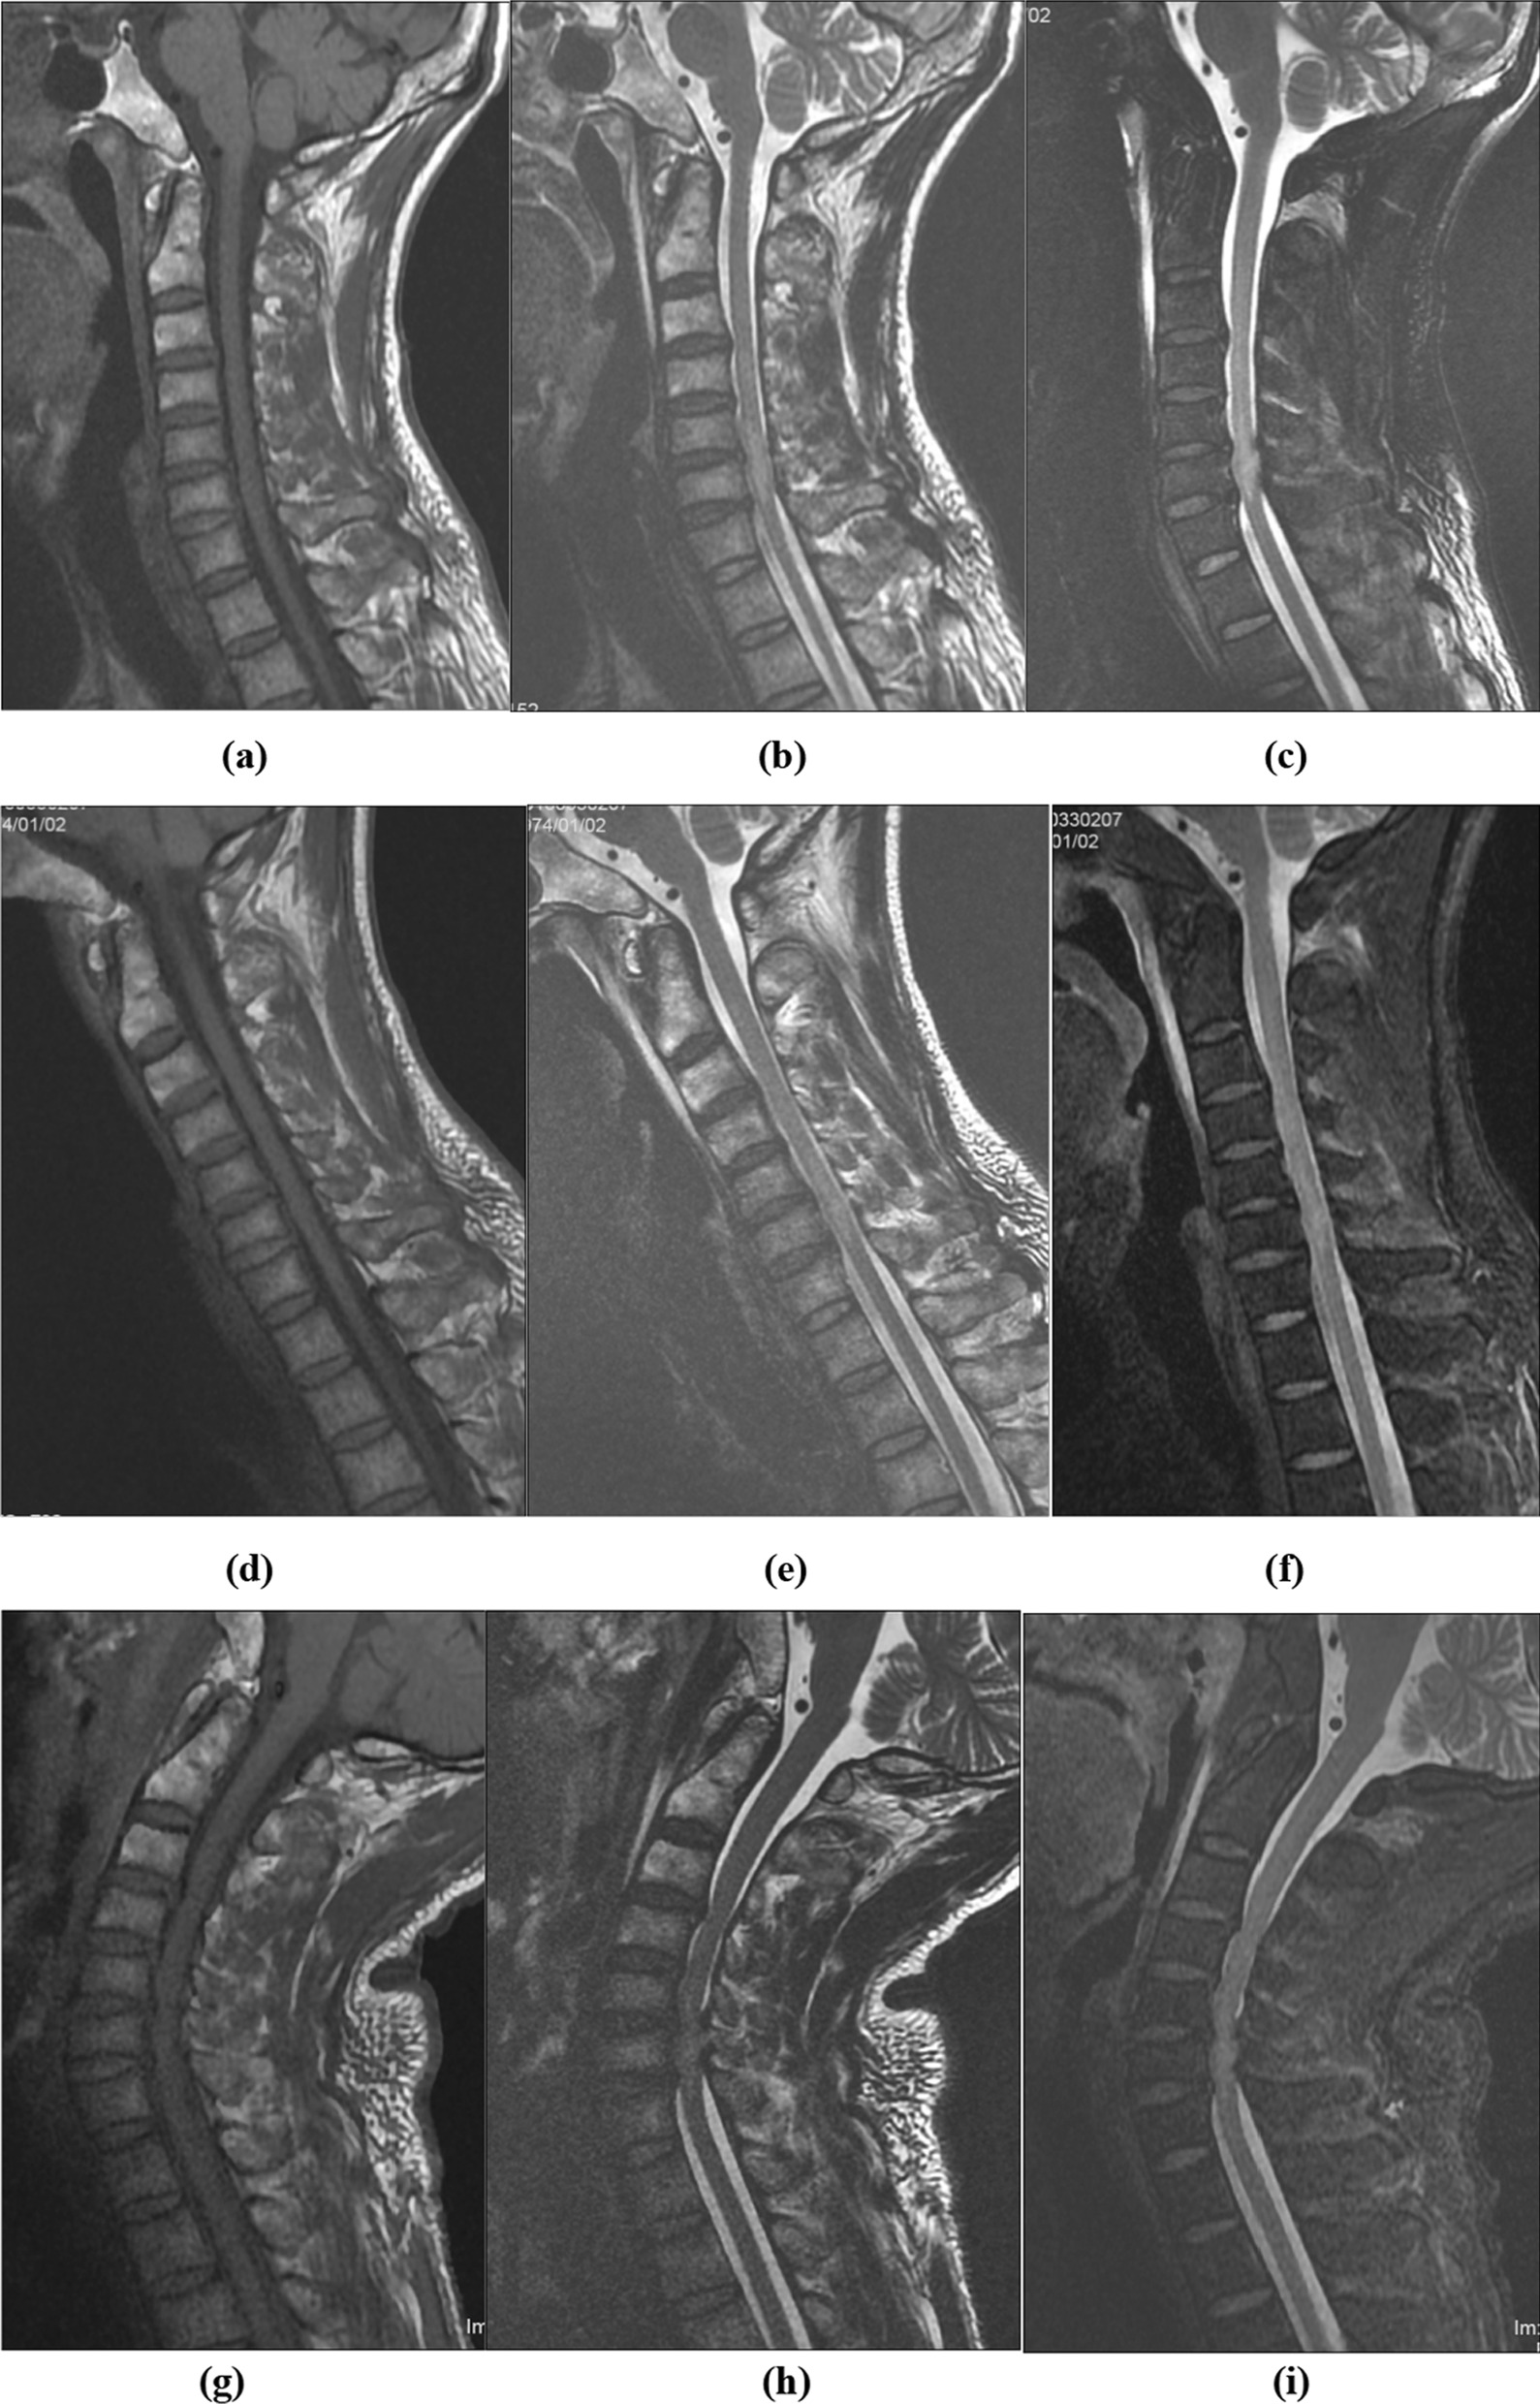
Cervical neutral sagittal T1-weighted MRI (a) confirmed the absence of vertebral body fracture and no signal of hemorrhage in the spinal cord of case 2; T2-weighted (b) and fat-saturated (c) imaging revealed abnormal signals of injury in the spinal cord from the C5 vertebral body to the cervical 6/7 disc level, in addition to disc herniation with spinal cord compression at C5/6 and C6/7. Cervical flexion MRI (d–f) showed reduced disc herniation without evident compression of the spinal cord at C5/6 and C6/7. Cervical extension MRI (g–i) showed aggravated disc herniation with spinal cord compression and canal stenosis at C5/6 and C6/7
